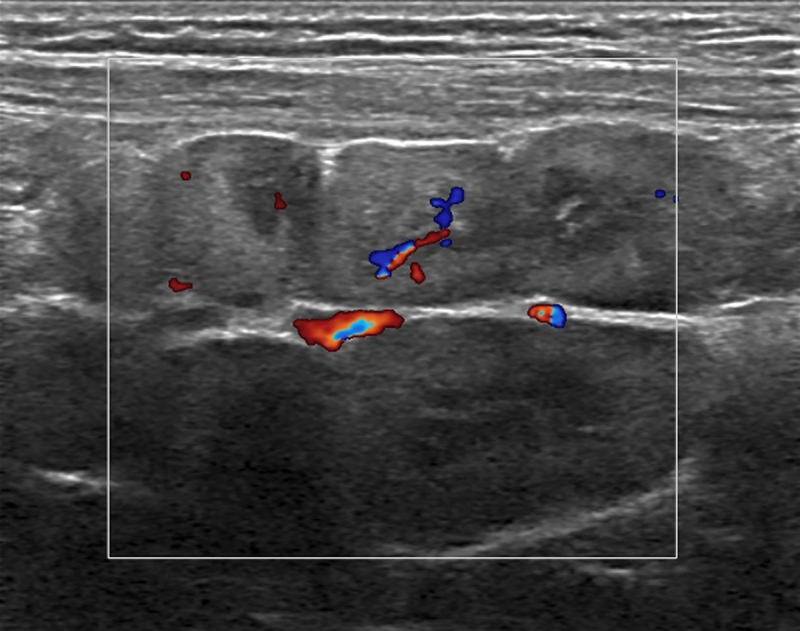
Colour Doppler ultrasound image demonstrating hilar vascularisation of the lymph nodes.
